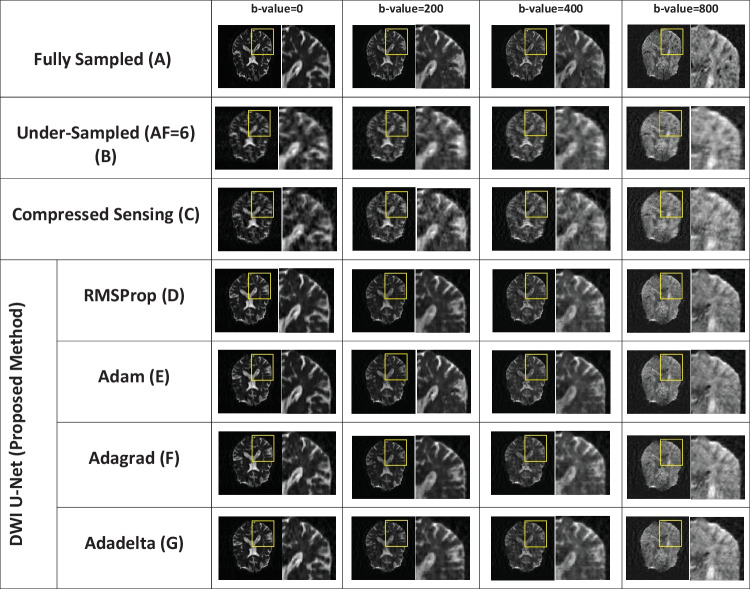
Reconstructed images of the human head OASIS dataset for the proposed method (DWI U-Net) with different optimizers, i.e., RMSProp, Adam, Adagrad, Adadelta, and Compressed Sensing at *AF* = 6 with different *b*-values, i.e., 0, 200, 400, and 800 s/mm^2^ (left to right in each column), respectively
